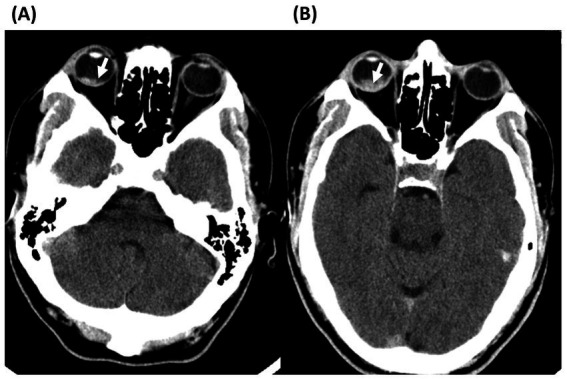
Axial head computed tomography without contrast of case 3. (A) CT head without contrast at initial presentation showing a spontaneous intraocular hemorrhage in the posterior pole of the right eye. (B) CT head after administration of intravenous thrombolysis with expansion of the hemorrhage.
